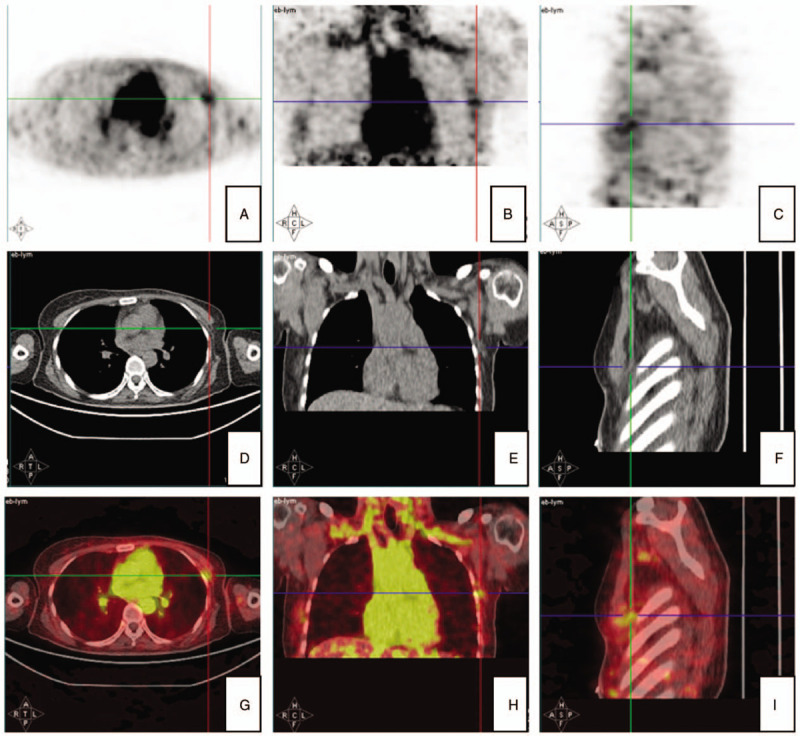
^68^Ga-NEB PET/CT enabled identification of the chylous fistula location on the inferior branch of the left subclavian lymph vessel after SLNB. Total general phase with delayed images indicated increasing tracer intensity at the left axilla from the (A) axial, (B) coronal, and (C) sagittal views. Lesion CT phase images showed the location of the fistula from (D) axial, (E) coronal, and (F) sagittal views. Lesion fusion phase images enabled fistula localization beyond the left subclavian vein, anterior to the left axillary vein from the (G) axial, (H) coronal, and (I) sagittal views.
